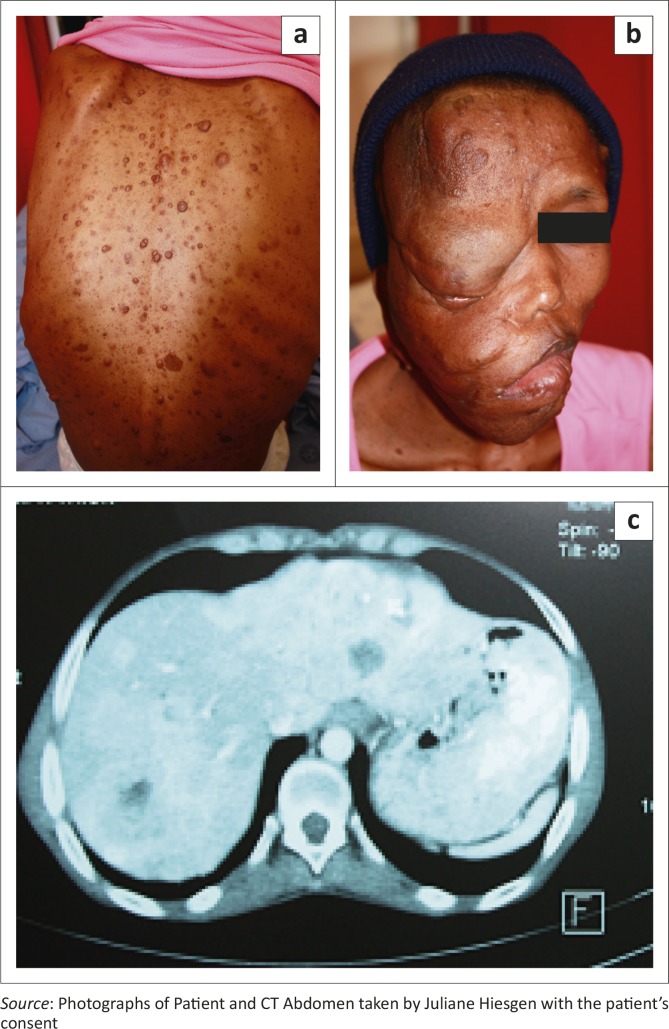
Clinical features of NF 1, with numerous neurofibromata (a), big plexiform neurofibroma over the right side of the face (b) and (c) CT image showing several hypodense lesions in the liver parenchyma, suggestive of metastases. *Source*: Photographs of Patient and CT Abdomen taken by Juliane Hiesgen with the patient's consent
